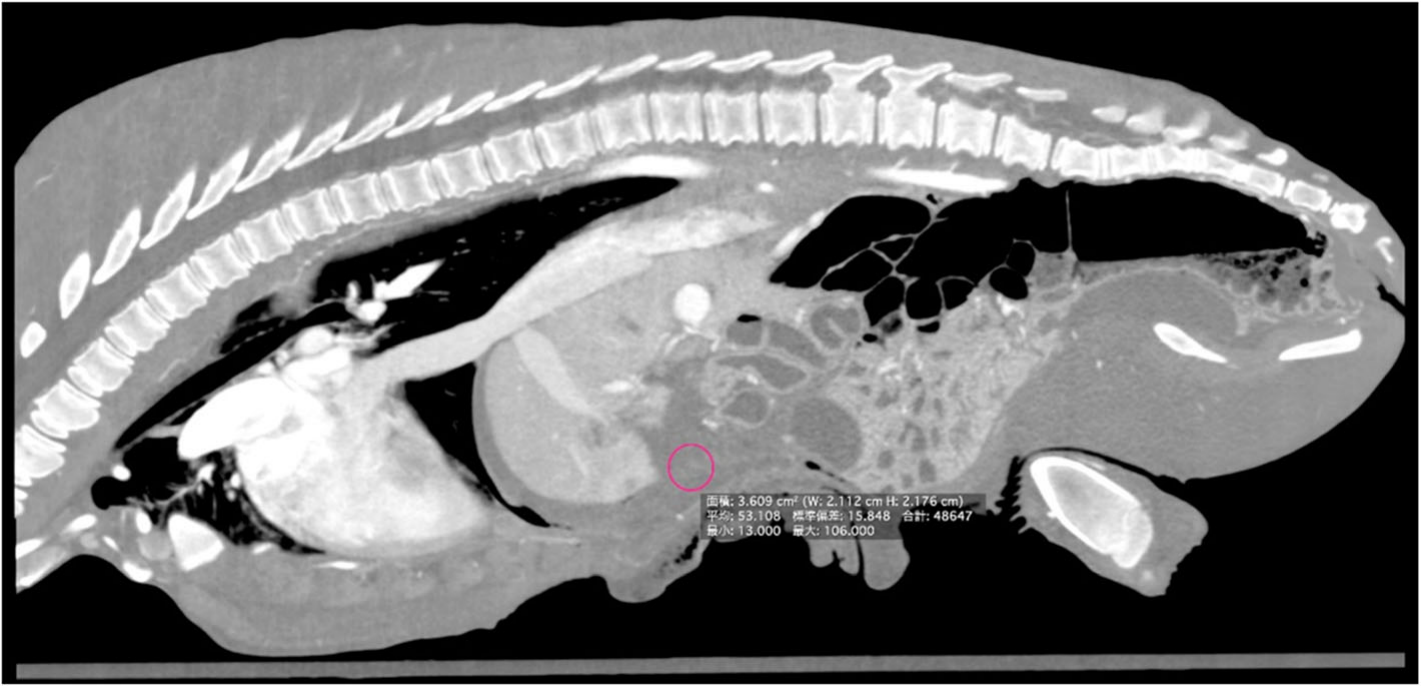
Sagittal CT image on the day following the surgery. The image depicts fluid accumulation, which appeared to be blood (CT value; 53 HU). CT, computed tomography; HU, Hounsfield units
